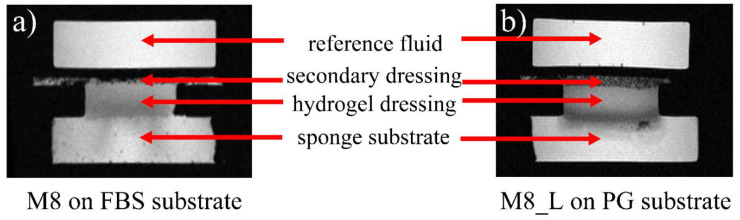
Images obtained at 20th echo (70 ms) at 15 min with MRI MSME sequence for M8 dressing samples on FBS wetted substrate (a) and M8_L dressing on PG wetted substrate (b).
